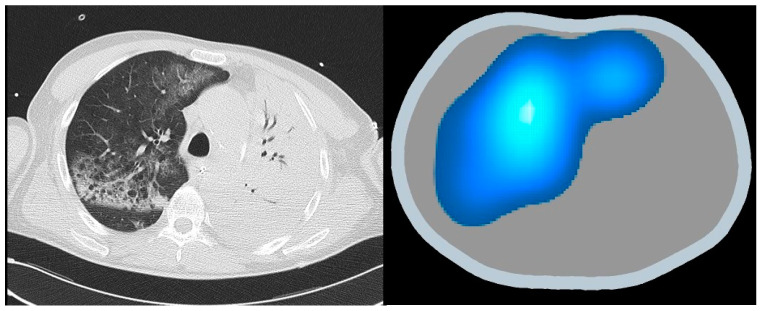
Revealing lung inhomogeneities in ARDS: comparing CT scan with EIT.
